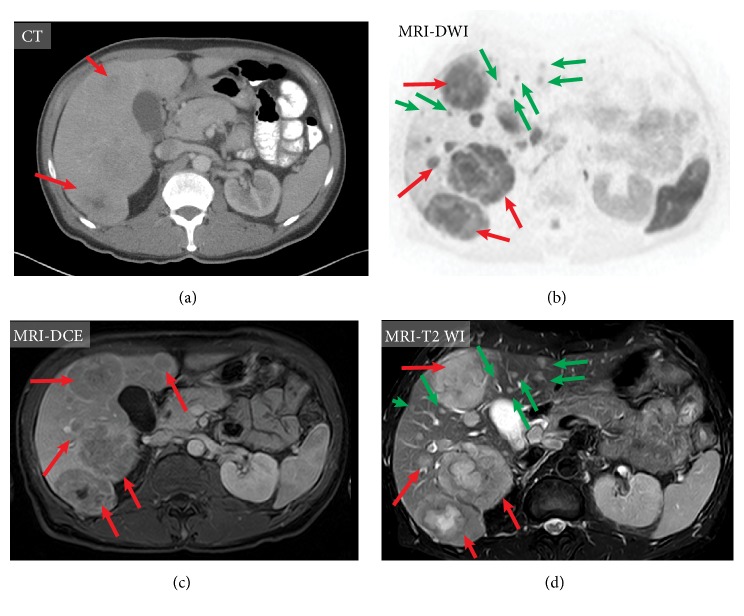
Imaging of hepatic metastases in medullary thyroid carcinoma of the same patient using contrast-enhanced computed tomography (CT) (a), magnetic resonance imaging (MRI), diffusion-weighted sequence (b); contrast-enhanced MRI, T1 weighted images (DCE)(c); MRI, T2 weighted image (d). MRI shows improved visualization of metastatic lesions compared to CT (red arrows) and can also detect very small metastases (green arrows), which may not be visible on CT.
